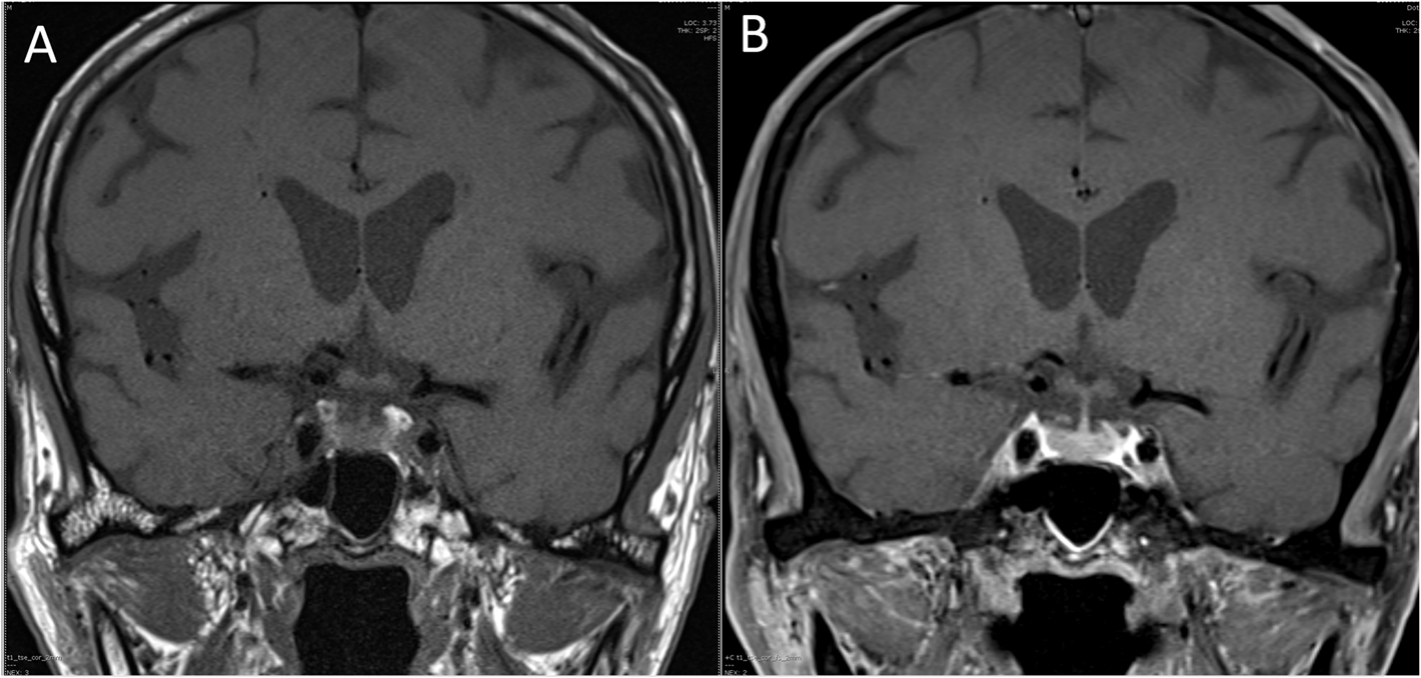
Magnetic resonance imaging (MRI) of the pituitary gland, coronal plane. a T1-weighted MRI showing no abnormalities in the pituitary gland, hypophyseal stalk, and hypothalamus. b Gadolinium-enhanced T1-weighted MRI showing a symmetric, round-shaped pituitary gland with homogeneous enhancement of the hypophyseal stalk
